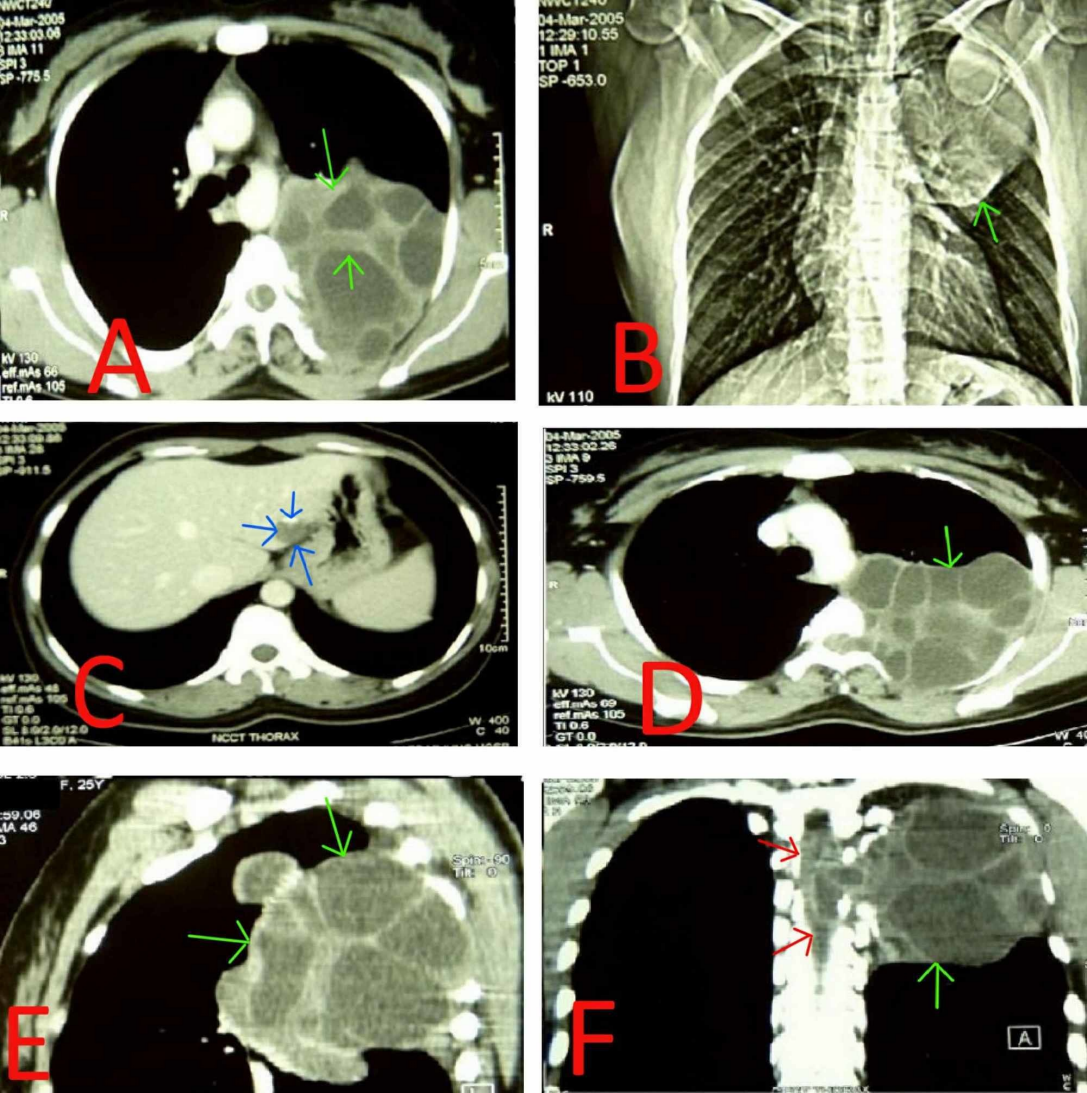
Noncontrast CT of chest and abdomen. (A) & (D) Shows a large, lobulated and septated cystic lesion (green arrows) in left para vertebral gutter. (B) Shows the superior inferior extent of the lesion, extending from just below the thoracic inlet to the level of the left atrium (green arrows). (C) Liver shows a non-enhancing, septated cystic lesion with eccentric enhancement in the medial segment of the left lobe of liver (blue arrows). Spleen, gall bladder, intra hepatic biliary radicals and kidney appear normal. (E) Shows the anterior-posterior extent of the lesion (green arrows). (F) Coronal plane view showing the lesion (green arrows) and the extension of the lesion (red arrows) in to the spinal canal hence causing neurological symptoms.
